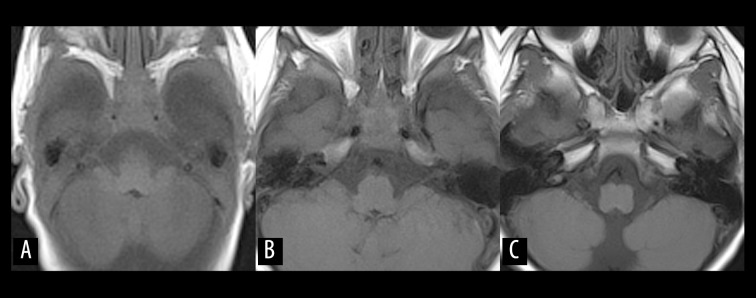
Normal fatty bone marrow infiltration of the skull base in childhood. (A–C) Non-contrast, T1-weighted images of a 3-month-old baby (A), and 2.5-year-old (B) and 5-year-old (C) girls demonstrate serial signal change from iso- to hyperintensity of the bone marrow at the skull base, corresponding to normal conversion from cellular to fatty marrow in childhood.
